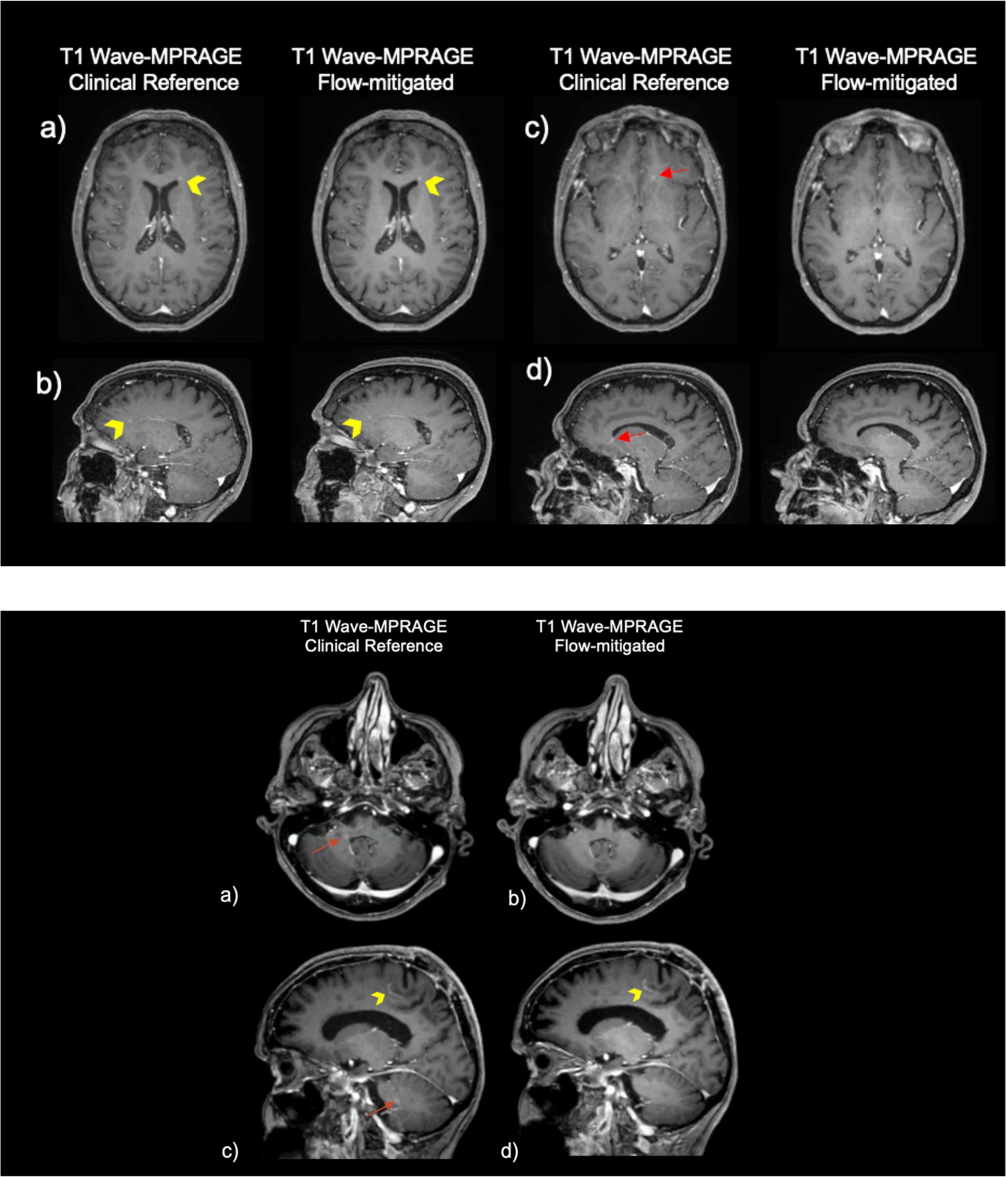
Two representative pathologic cases with true enhancing lesions as well as flow-related artifacts mimicking additional enhancing lesions. The flow-mitigated technique was able to discern true enhancing lesions from the flow-related artifact, which were absent on flow-mitigated post-contrast T1-weighted MPRAGE (magnetization-prepared rapid gradient-echo) images. Flow mitigation improved diagnostic accuracy in both cases and more accurately assessed the degree of disease burden. Patient 1 (P1): 71-year-old female with stage 4 breast cancer who underwent contrast-enhanced brain MRI to screen for intracranial metastatic disease. Axial (a) and sagittal (b) images show a 3-mm enhancing focus in the left forceps minor (arrowhead) on the conventional and flow-mitigated Wave-MPRAGE images, consistent with a metastatic lesion, demonstrating true *versus* false enhancing focuses on T1-weighted Wave-MPRAGE images. Axial (c) and sagittal (d) views show a punctate focus of apparent enhancement in the left inferior frontal lobe on the conventional Wave-MPRAGE images that is not present on the corresponding flow-mitigated Wave-MPRAGE images, suggesting the area of enhancement was artifactual. Patient 2 (P2): 82-year-old female with progressive multifocal leukoencephalopathy showing a small focus of apparent enhancement (arrows) on axial (a) and sagittal (c) views of conventional T1-weighted post-contrast Wave-MPRAGE imaging. No enhancement was noted on the corresponding axial (b) and (d) sagittal flow-mitigated Wave-MPRAGE images, suggesting that the area of apparent enhancement was consistent with flow-related artifact. Curvilinear enhancement is also seen in the posterior frontal lobe subcortical U-fiber (arrowhead), corresponding to a white matter demyelinating lesion associated with the patient’s known diagnosis of progressive multifocal leukoencephalopathy
